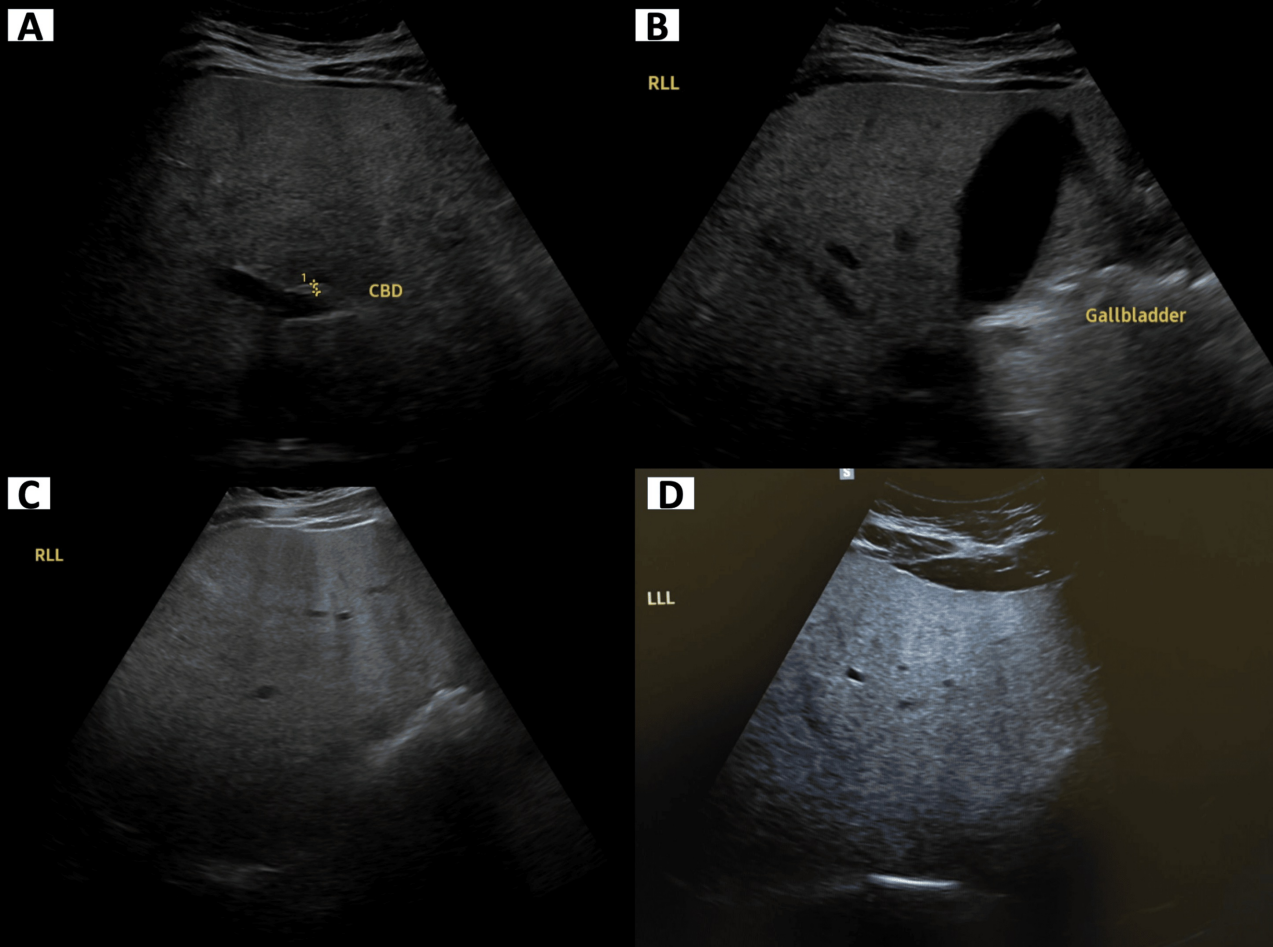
Abdominal ultrasound demonstrating diffusely increased hepatic echogenicity consistent with hepatic steatosis. (A) Common bile duct (CBD) with normal caliber. (B) Gallbladder with no evidence of cholelithiasis or cholecystitis. (C) Right lobe of the liver demonstrating increased parenchymal echogenicity consistent with diffuse hepatic steatosis. (D) Left lobe of the liver showing increased echogenicity consistent with fatty infiltration.
